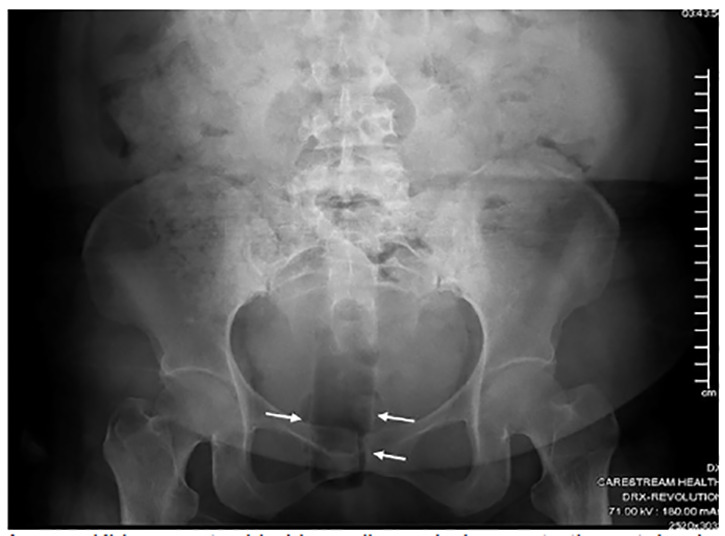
Kidney-ureter-bladder radiograph demonstrating retained rectal foreign body as lucency in rectum (arrows).
